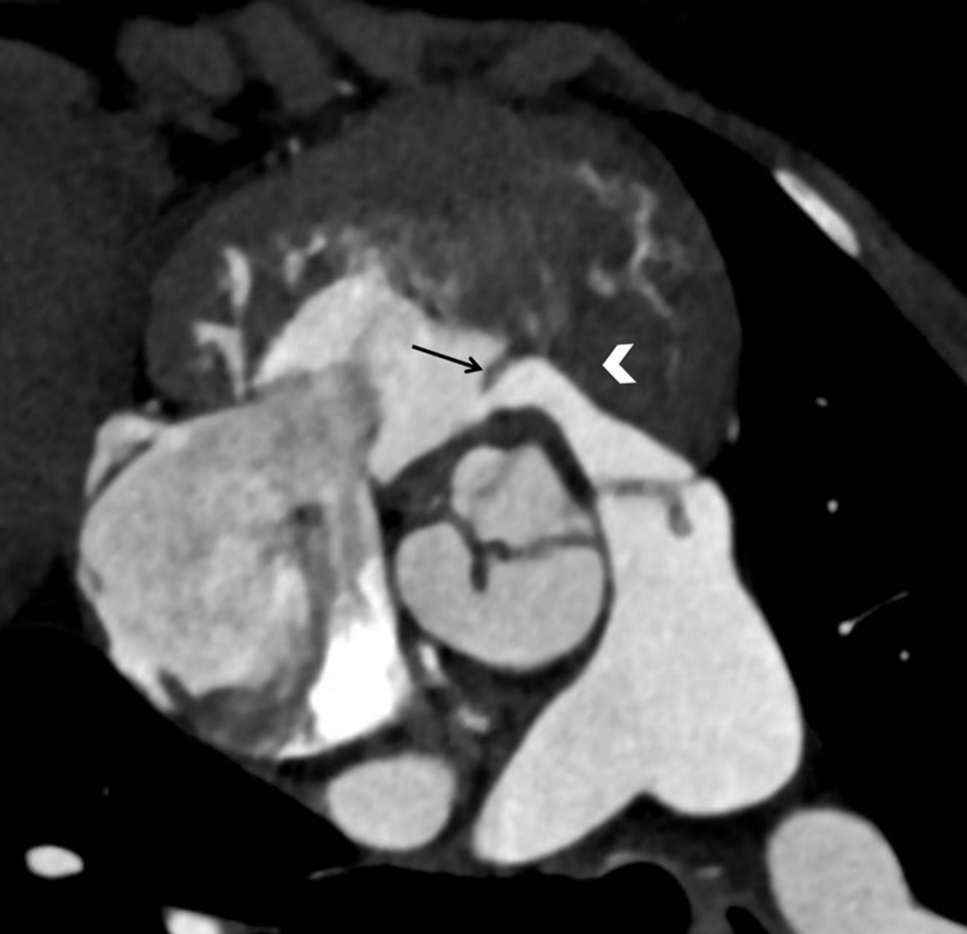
Cardiac CT short axis RVOT reformat showing thickened and hypertrophied infundibulum (chevron) with an anomalous band (thin arrow) running beneath the infundibulum of the outflow tract
